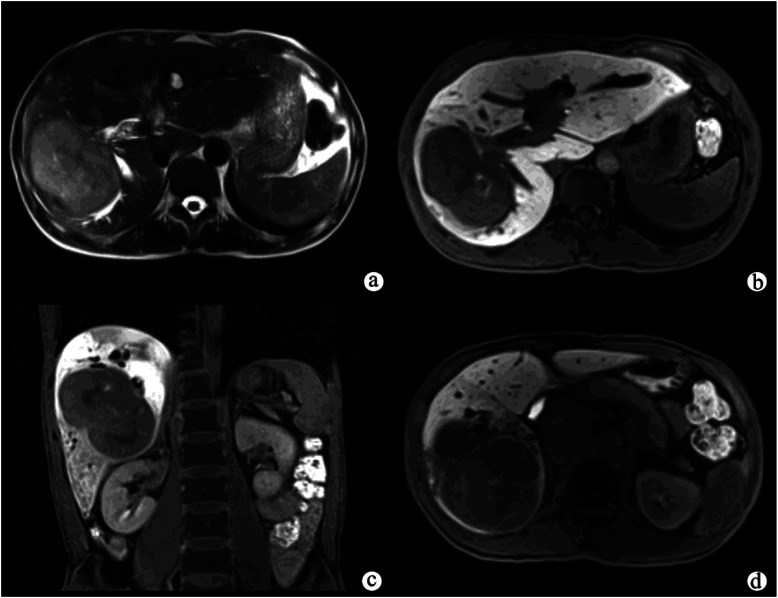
Exophytic peripheral cholangiocarcinoma in a patient with abdominal pain. A T2-weighted image (a) showed a large, hyperintense mass with lobulated margins in the right hepatic lobe, and the adjacent bile duct was dilated. The hepatocellular phase (b, c) showed that the tumor was inhomogeneously hypointense compared with the liver parenchyma. The liver parenchyma also showed inhomogeneous enhancement on the hepatocellular phase (c). The biliary phase (d) showed that the common bile duct was completely filled with Gd-EOB-DTPA, indicating that the function of biliary system was normal.
